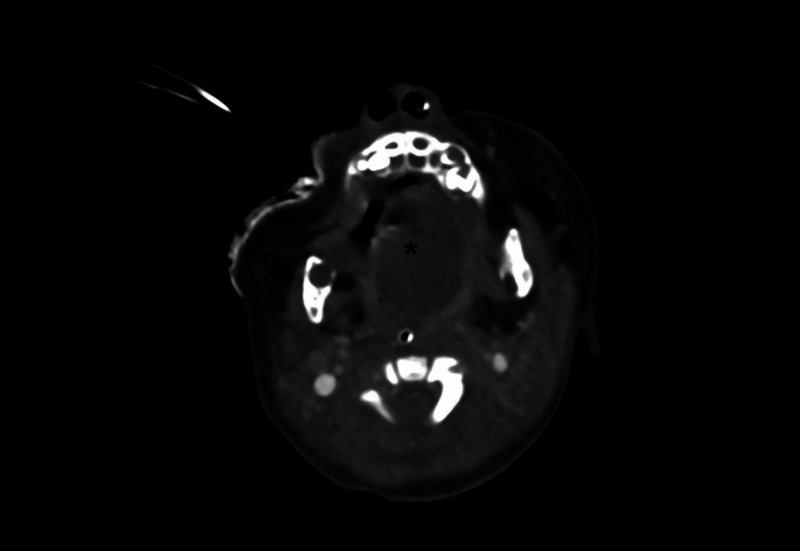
Axial CT scan of the neck with contrast showing mild peripheral rim enhancement measuring almost 3.4 x 2.5 x 2.2 cm (asterisk)
